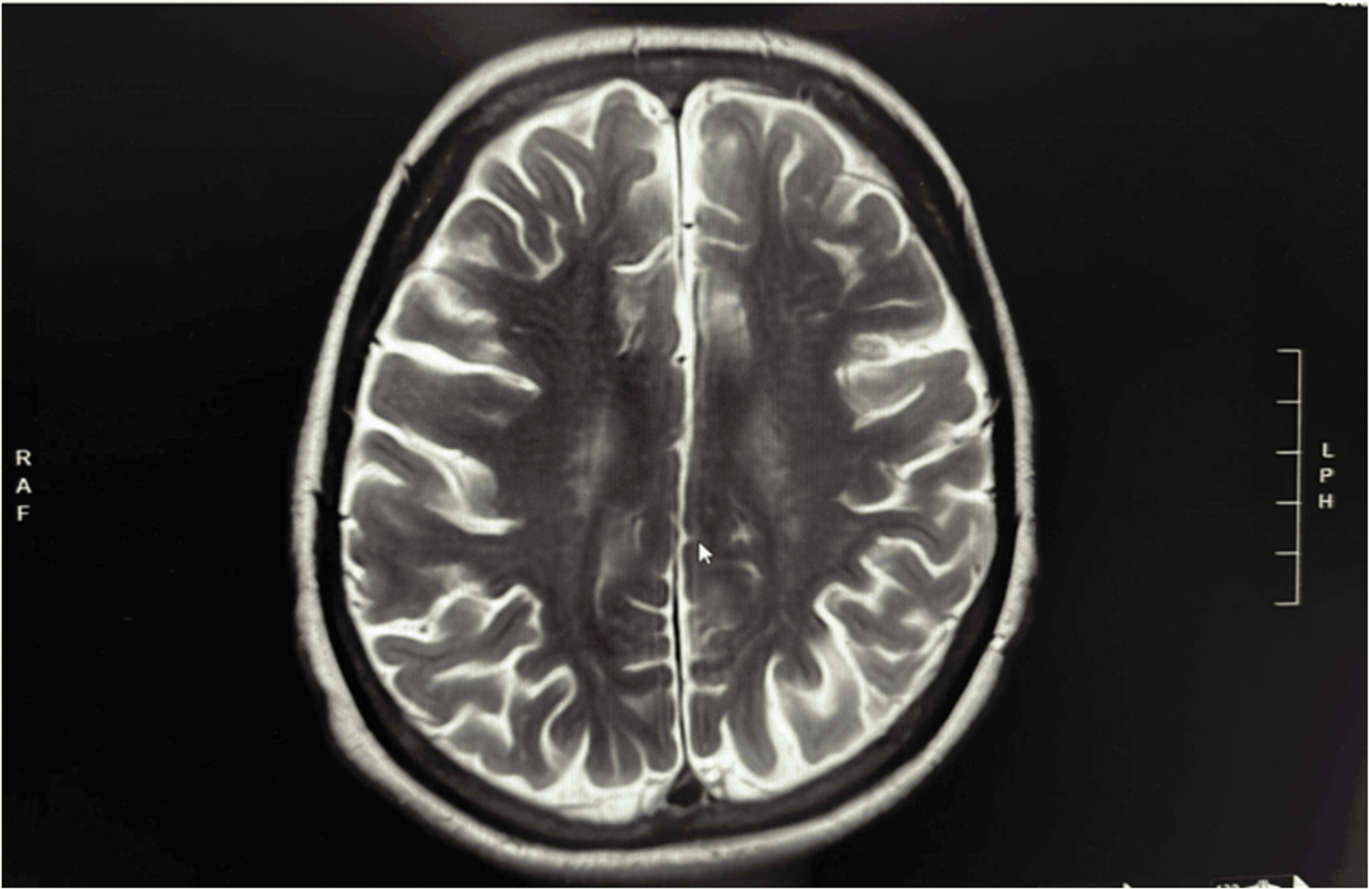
Contrast-enhanced MRI of the brain demonstrates mild chronic small vessel ischemic changes without evidence of acute infarction, parenchymal lesions, or contrast-enhancing masses, effectively ruling out intracranial metastasis.
